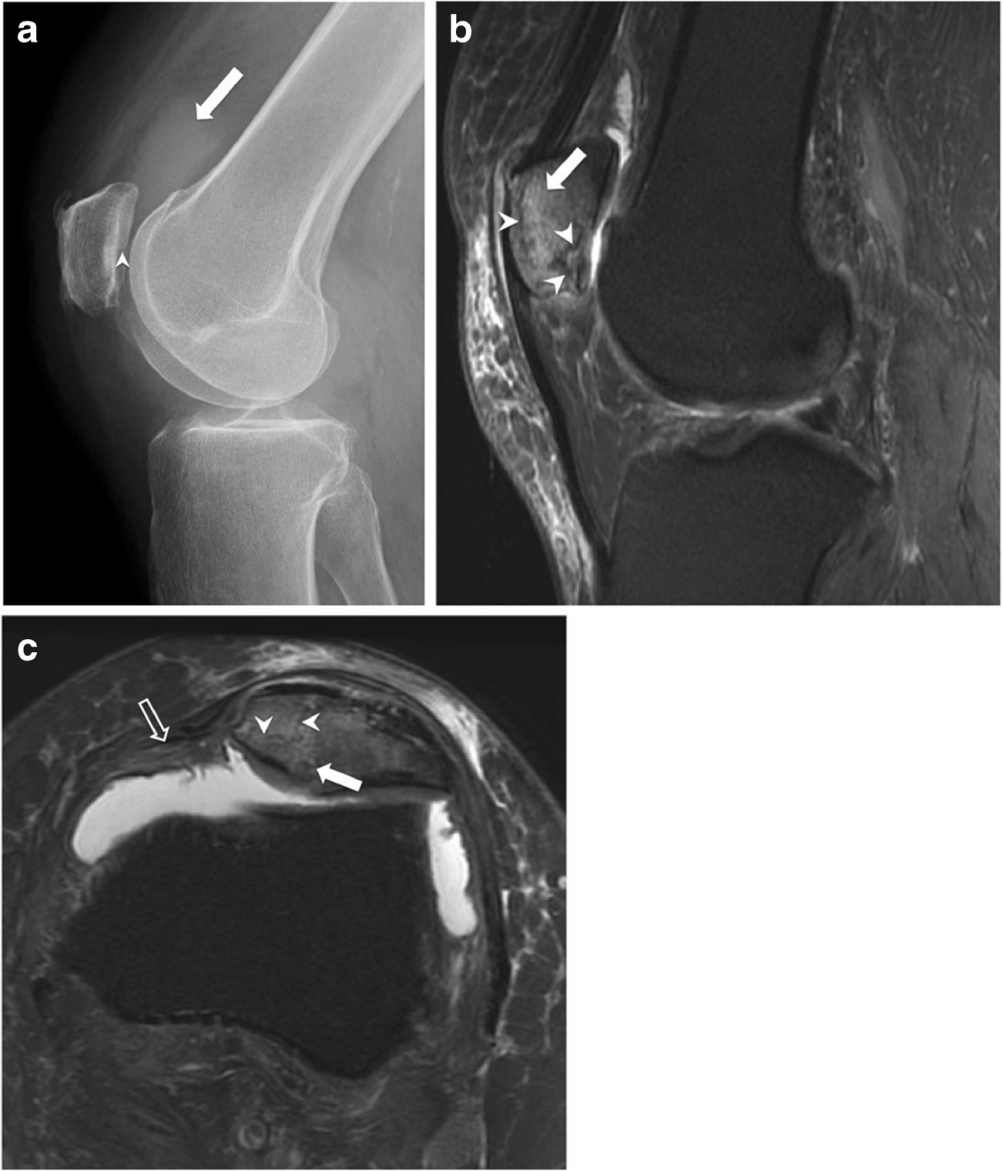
Stellate fracture with retinacular injury in a 40-year-old woman with persistent anterior knee pain for 2 weeks ago after a fall. (a) Lateral knee radiograph shows joint effusion (*arrow*) and a subtle linear lucency through the patellar undersurface (arrowhead), suggesting fracture. (b) Sagittal and (c) axial fat-suppressed proton density MR images reveal several fracture lines (*arrowheads*) consistent with stellate fracture, bone marrow oedema (*solid arrow*), and partial thickness tear of the medial retinaculum from its patellar attachment (*open arrow*)
